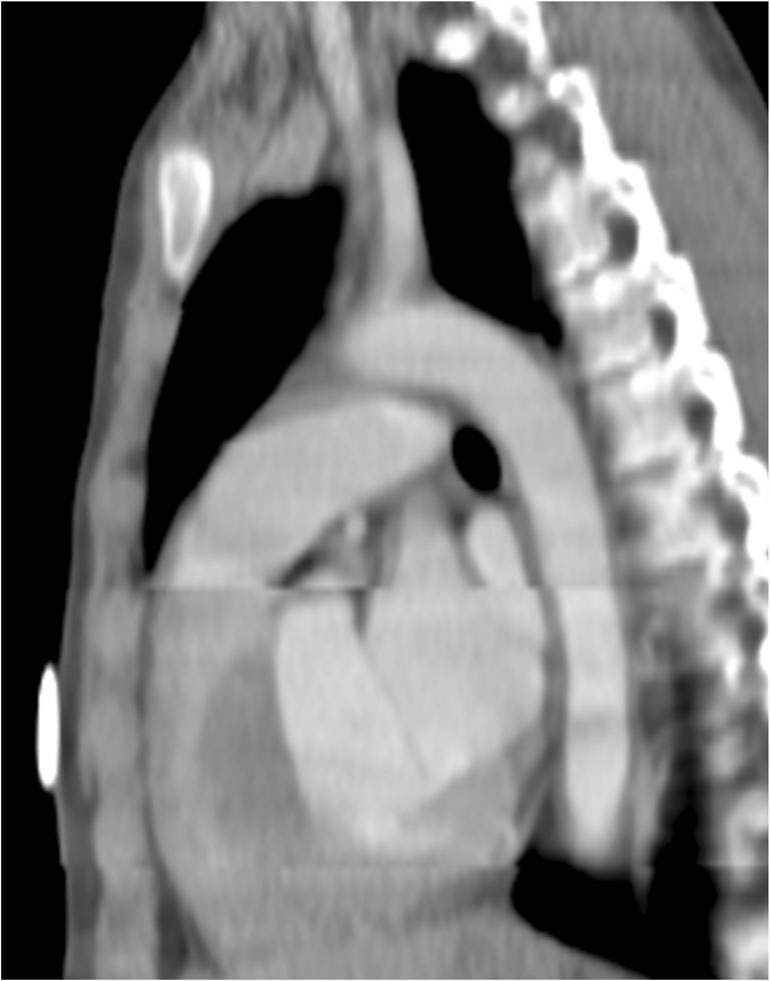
Coronal reformat from a thoracic CTA in a 13-year-old patient with mid aortic syndrome demonstrates step ladder artifact in the pulmonary artery as well as descending aorta. Note the differential enhancement in the right ventricle outflow tract vs. main pulmonary trunk. Also note the differential enhancement in descending aorta
